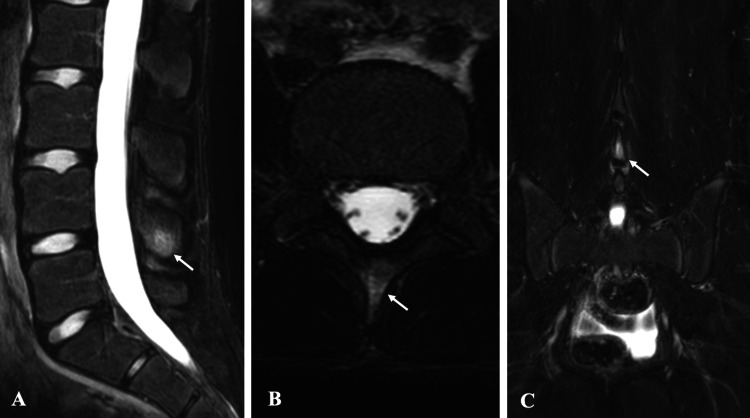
Pre-treatment MRI findings in Case 1. STIR sequence MRI reveals high signal intensity at the caudal aspect of the L4 spinous process: (A) sagittal view, (B) axial view, and (C) coronal view. MRI, magnetic resonance imaging; STIR, short-tau inversion recovery
